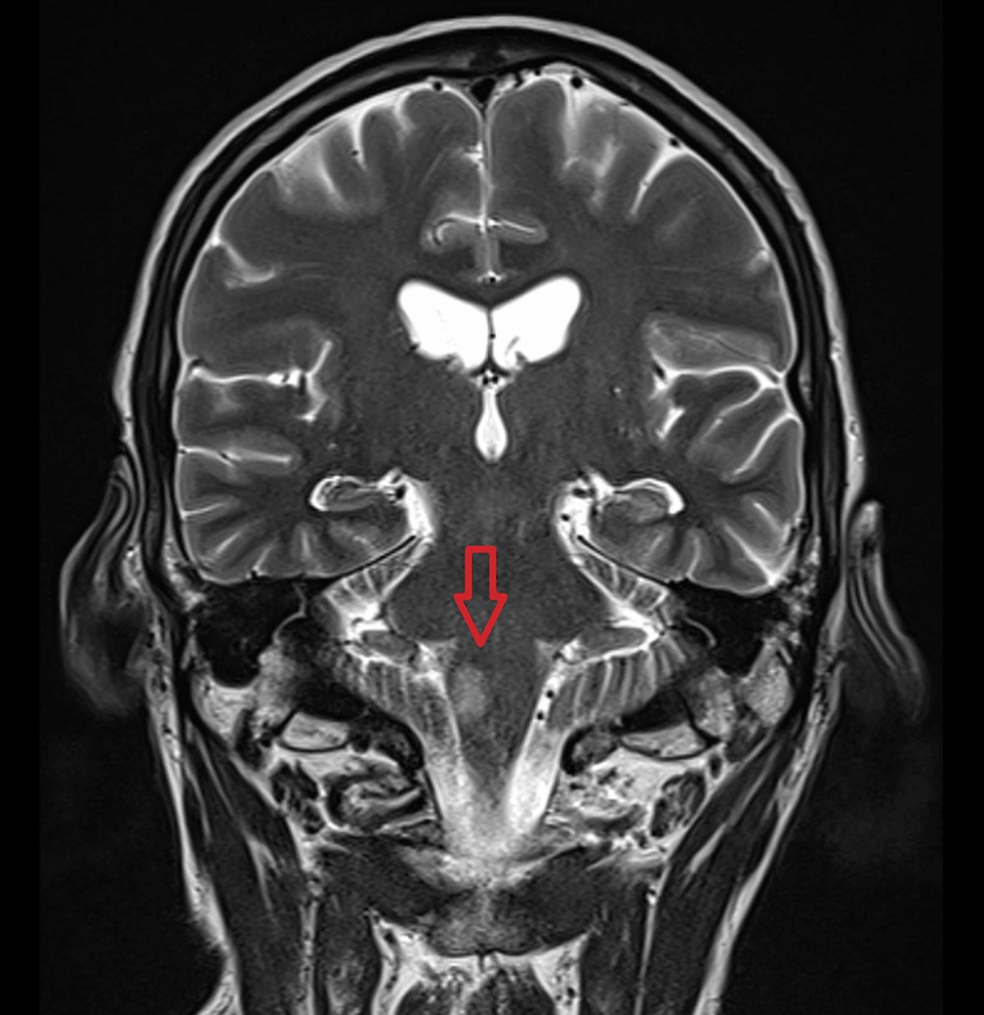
Coronal brain MRI with posterior circulation infarct in the right medulla oblongata
